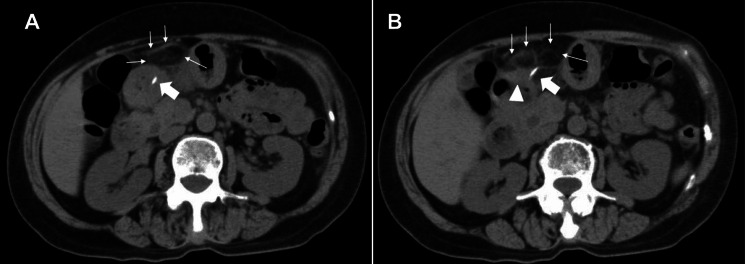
Multidetector computed tomography (MDCT) images of a fish bone-like structure and extra-gastric fat stranding showing inflammation of the stomach wall The marks included in this figure are as follows: Thick Arrow: a linear fish-bone-like abnormality extending from the anterior wall of the gastric antrum (A) to the outside of the gastric wall (B), representing a high-density structure; Thin Arrow (A, B): thickening of the stomach wall in the antrum; Triangle (B): extra-gastric fat stranding.
